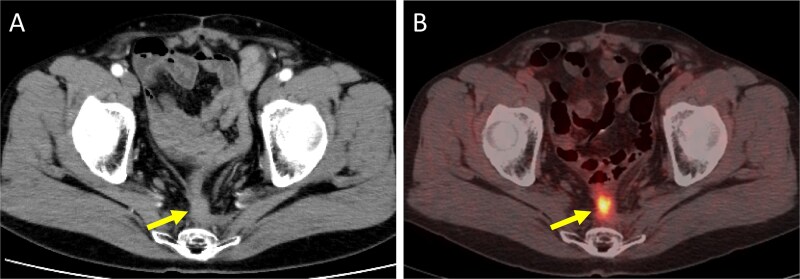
Imaging findings at the time of local recurrence diagnosis. (A) Contrast-enhanced CT. (B) Positron emission tomography-CT. Arrows indicate the recurrent lesion.
